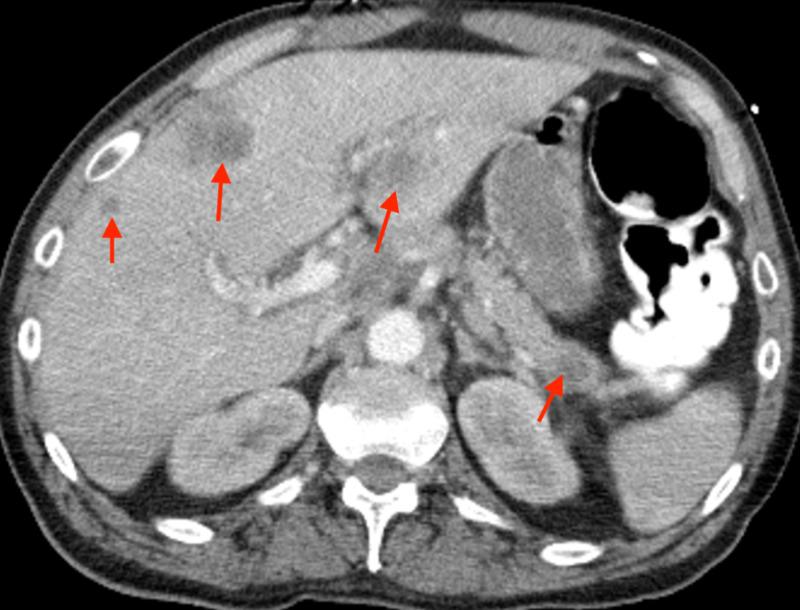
CT of the abdomen with contrast The study reveals diffuse metastatic disease found throughout the liver and a heterogeneous mass with areas of necrosis at the distal body and tail of the pancreas. These findings are consistent with a metastatic pancreatic carcinoma.
